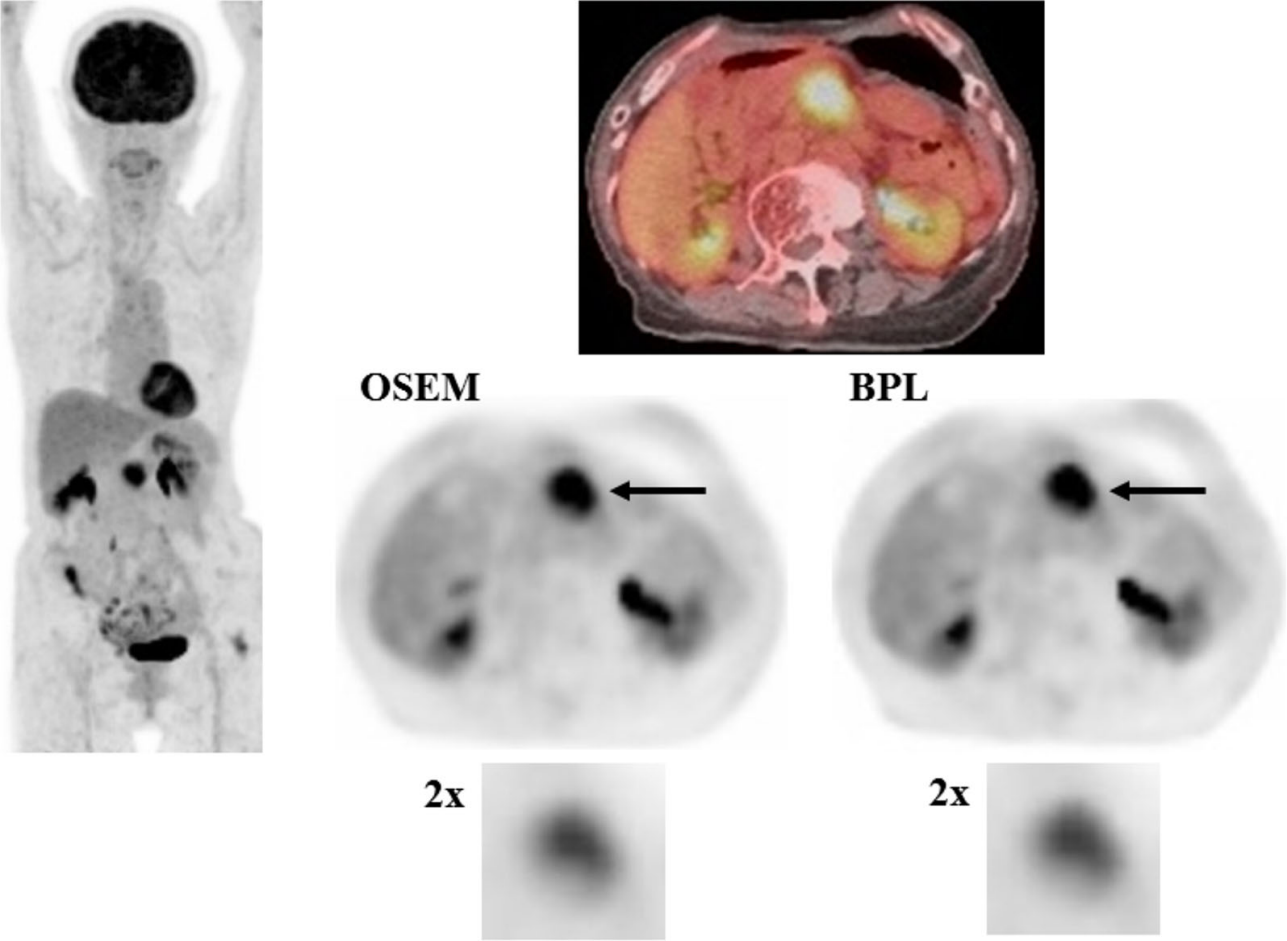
A case with pancreatic cancer (larger lesion). FDG uptake corresponding to the pancreatic cancer lesion (arrow) was visualized almost equally in OSEM and BPL images (right, middle and lower). SUVmax in OSEM (9.3) was similar to that in BPL image (9.2), and MTV was smaller in OSEM (10.3 ml) than in BPL image (10.9 ml). BPL increased high gray-level zone emphasis by 7.6% and decreased low gray-level zone emphasis by 33.0% compared to OSEM. (Left: maximum intensity projection image of FDG PET; right upper: fused FDG PET/CT image; right middle: FDG PET images reconstructed with OSEM and BPL algorithms; right lower: images focusing on tumor FDG uptake magnified by a factor of 2).
